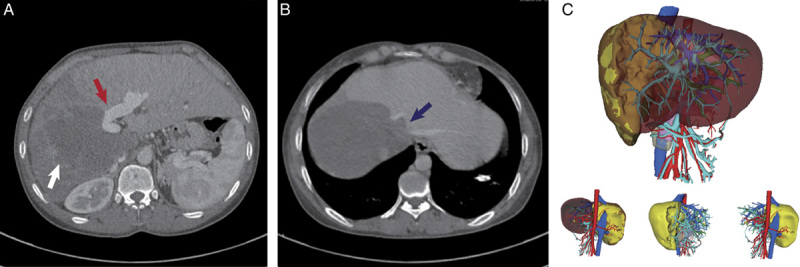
The preoperative imaging assessment and surgical procedures of ‘remnant-liver-first’ strategy for IRAT in patients (case 3) with end-stage HAE. (A) and (B) Preoperative CT demonstrated a giant lesion occupying the entire right liver and left medial section of the liver (white arrow) that invaded portal veins (red arrow) and retrohepatic inferior vena cava (blue arrow); (C) The three-dimensional reconstruction of the lesion, which provided information of remnant liver volume and visualized the vascular and biliary tract anatomy. IRAT, *in vivo* liver resection combined with autotransplantation; HAE, hepatic alveolar echinococcosis; CT, computed tomography.
